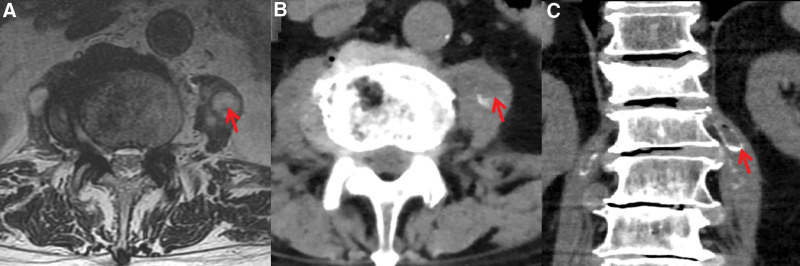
Imaging examination of lumbar vertebras. (A) MRI of cervical in horizontal view. (B) CT of cervical in horizontal view. (C) CT of cervical in coronal view. Red arrows indicated the psoas lesions. CT = computerized tomography scan, MRI = magnetic resonance imaging.
